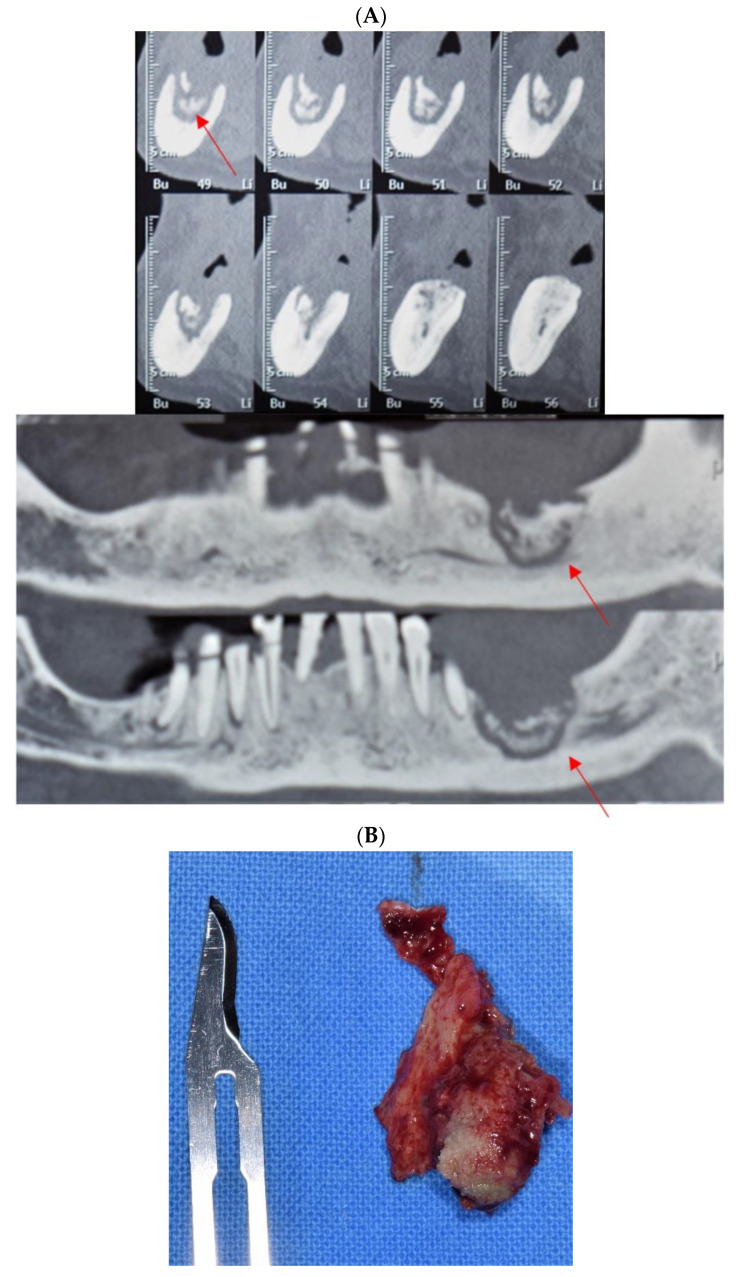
Clinical case of MRONJ localized in left mandible. (A) Cone beam computed tomography (CBCT) images, sagittal and frontal views, showing isolation of mandibular bone sequestration following antibiotic and topical antiseptic therapy (red arrows indicate the bone sequestrum). (B) Necrotic bone sequestration, resulting from the surgical intervention.
